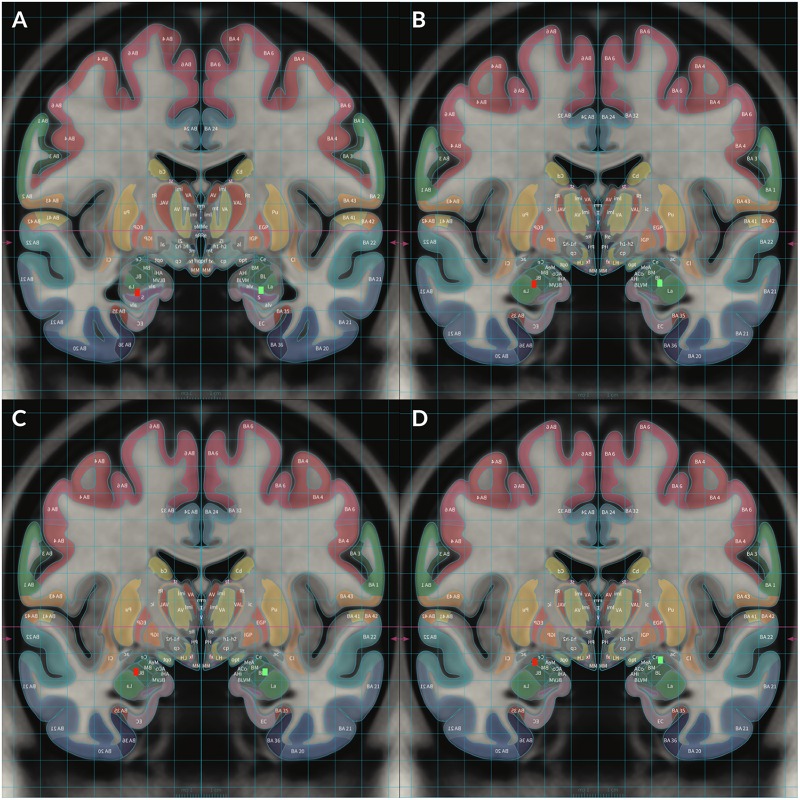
To demonstrate locations of each electrode, Subject 2’s MRI brain imaging studies were registered to the MNI space (MNI152 NLIN2009a) and then overlaid on the structural atlas of Mai (Horn and Kühn, 2015; Mai and Majtanik, 2017) to allow delineation of cerebral cortices and subcortical structures with color coded representations of cortical and subcortical parcelation according to the Mai atlas. The red rectangular markers represent right sided DBS electrode contacts 8–11 while the green rectangular markers represent left sided contacts 0–3. Contact 0 was placed in the left HC and contact 8 was placed in the right HC (A). Contact 1 was placed in the left ventral BLA and contact 9 was placed in the right ventral BLA (B). Contact 2 was placed in the left dorsal BLA and contact 10 was placed in the right dorsal BLA (C). Contact 3 was placed in the left CeA and contact 11 was placed in the right CeA (D).
